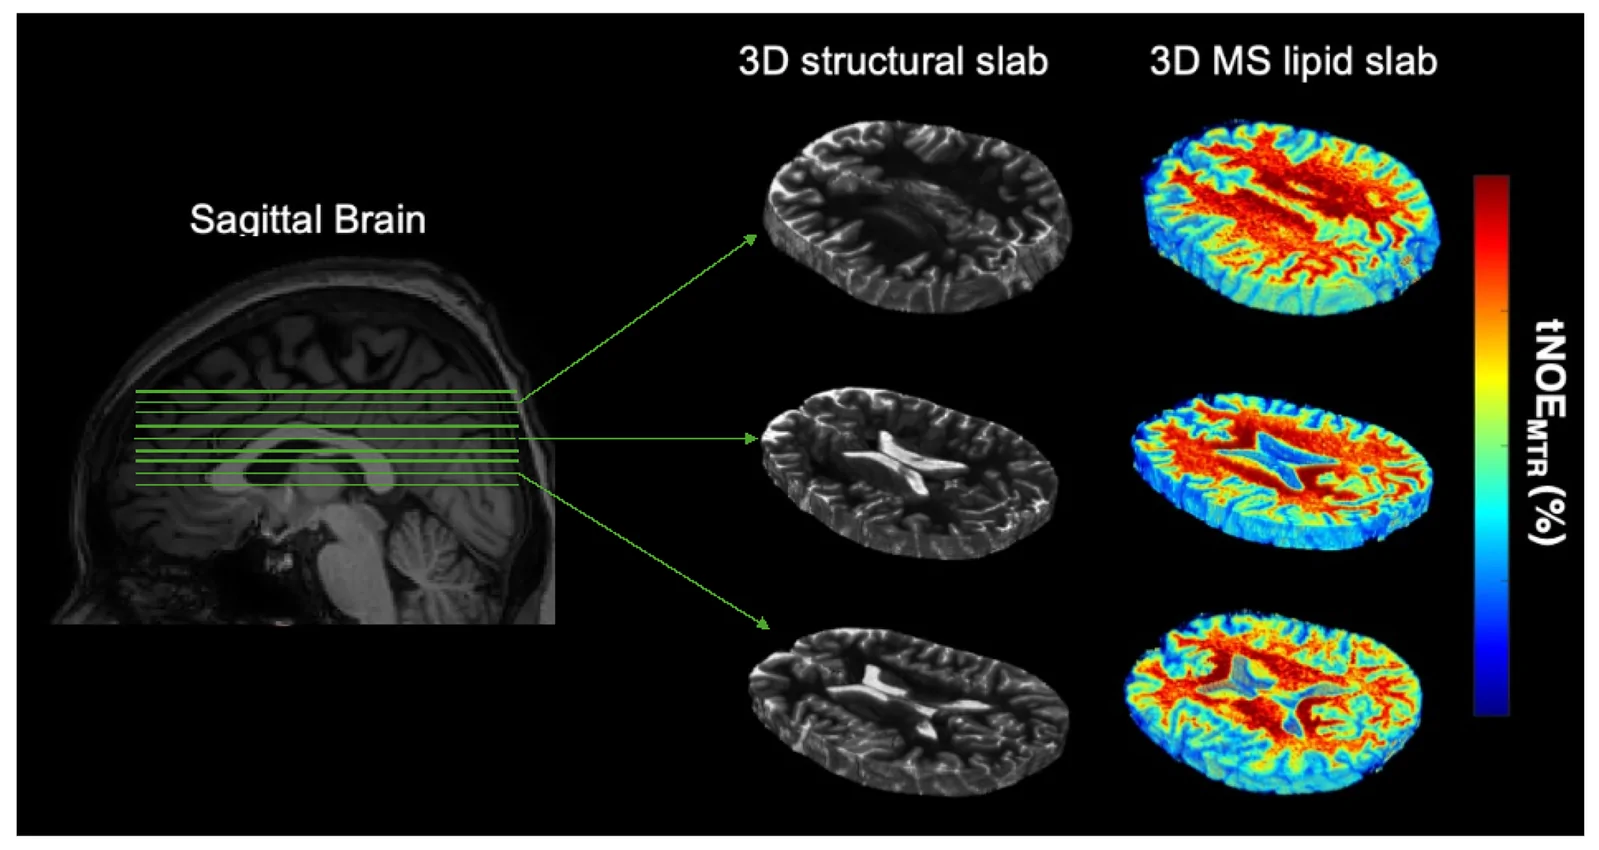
Representative 3D structural and lipid-sensitive tNOE imaging acquired through the corpus callosum in an MS subject. The left panel shows a sagittal T1-weighted brain image with green lines indicating the position and coverage of the partial 3D imaging slab. Corresponding slices from the 3D structural slab (middle column) and lipid-sensitive tNOE maps (right column) are displayed. The tNOE maps, acquired using the optimized adiabatic preparation, demonstrate strong contrast between white matter and surrounding tissues, highlighting regional variations in lipid-related signal across the corpus callosum and adjacent white matter structures. The color bar represents tNOE_MTR_ contrast (%).
